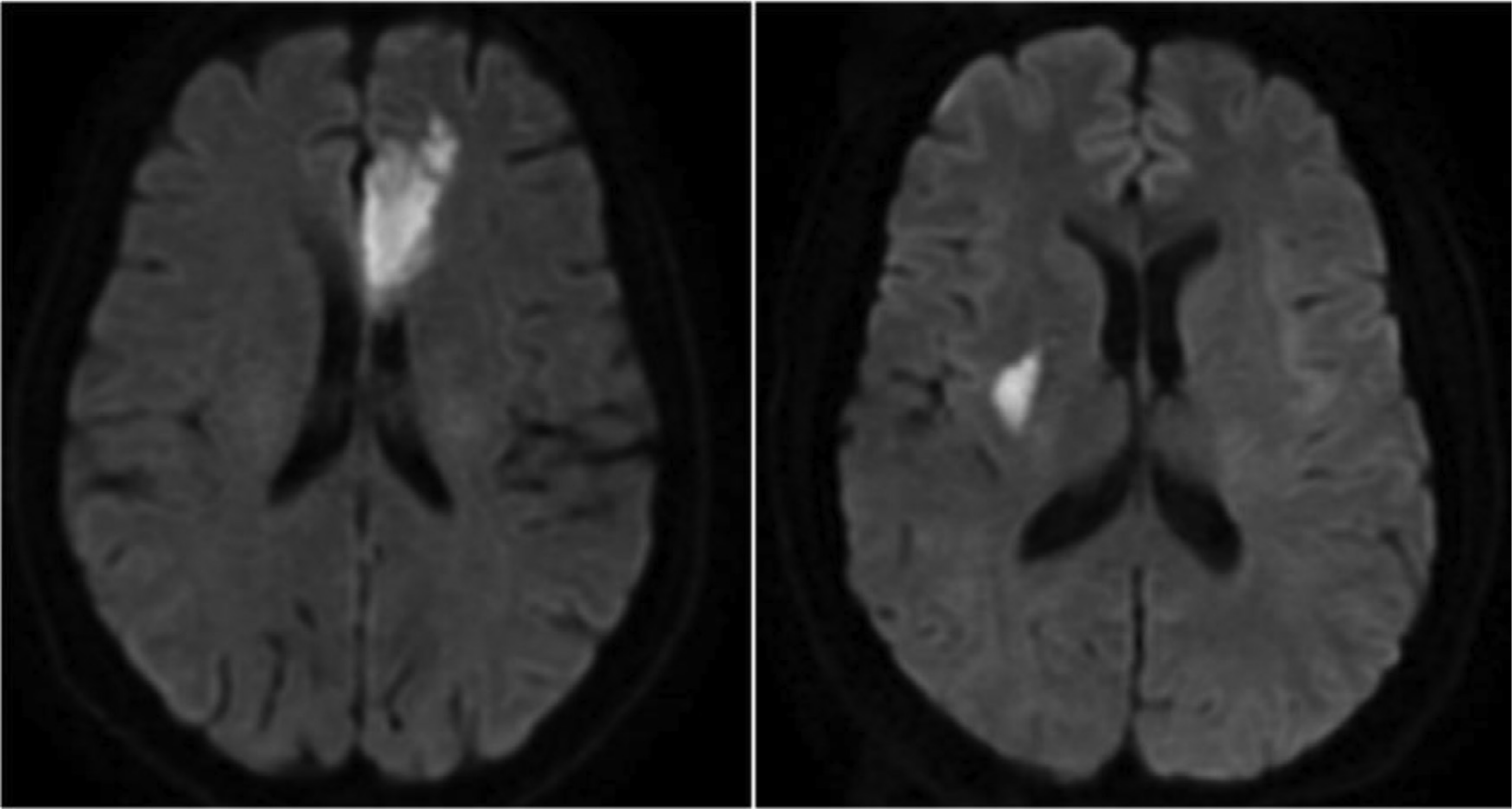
The acute infarctions of two patients of PSD on DWI. Left: A 63-year-old female, she was admitted because of right limb weakness and aphagia with NIHSS score 3. The brain MRI showed acute infarction in left frontal lobe. After hospitalization for 7 days, she was discharged with a NIHSS score 2. Three months later she had no obvious functional impairment but had a GDS score 9 and was identified to have PSD. Right: A 54-year-old male, he was admitted because of weakness and numbness of left limb with NIHSS score 3. The brain MRI showed acute lacunar infarction in right basal ganglia. During hospitalization he didn't react well to the treatment and had neurological deterioration with NIHSS score 5 at the 7^th^ day of hospitalization. Three months later he had a GDS score 10 and was identified to have PSD.
